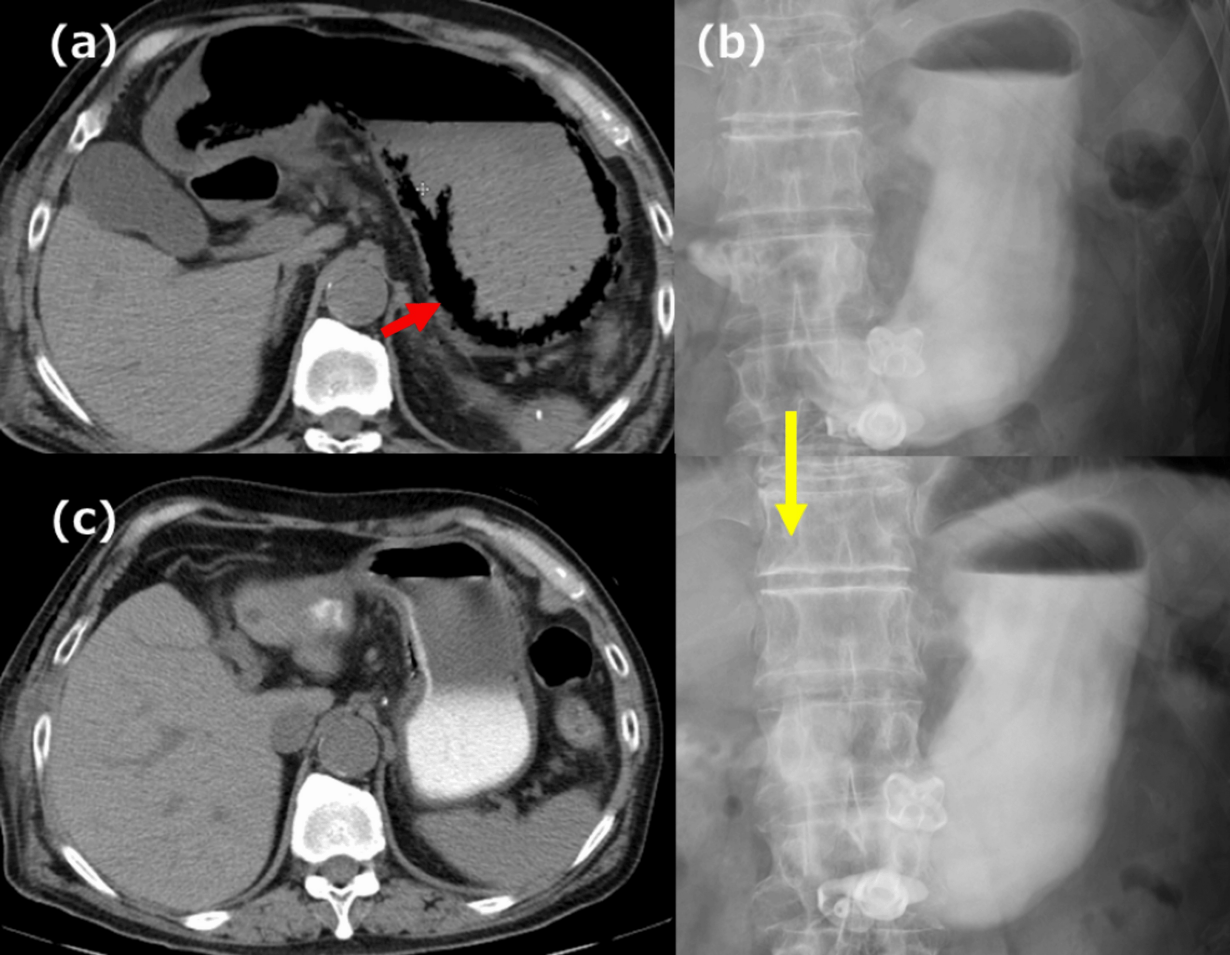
CT and upper GI series findings in Case 2 (a) CT showed gastric emphysema (red arrow). (b) Four hours later, Gastrografin🄬 iodinated contrast did not pass the pylorus, indicating DGE. (c) After the sixth day of conservative treatment, the gastric emphysema had resolved. CT: computed tomography; GI: gastrointestinal; DGE: delayed gastric emptying
